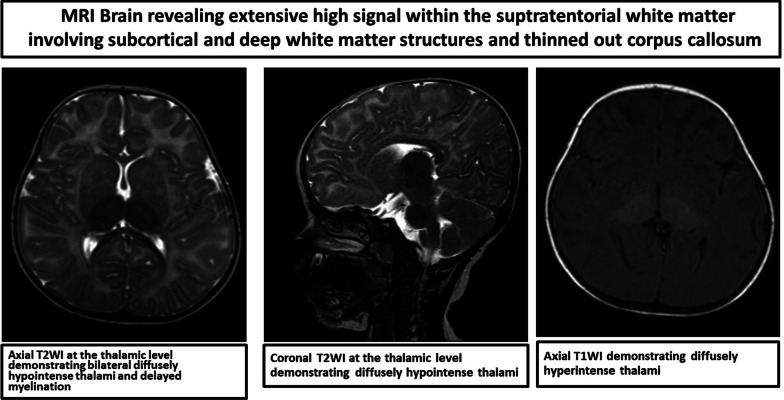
Magnetic resonance imaging of brain revealing extensive high signal within the supratentorial white matter involving subcortical and deep white matter structures and thinned-out corpus callosum
